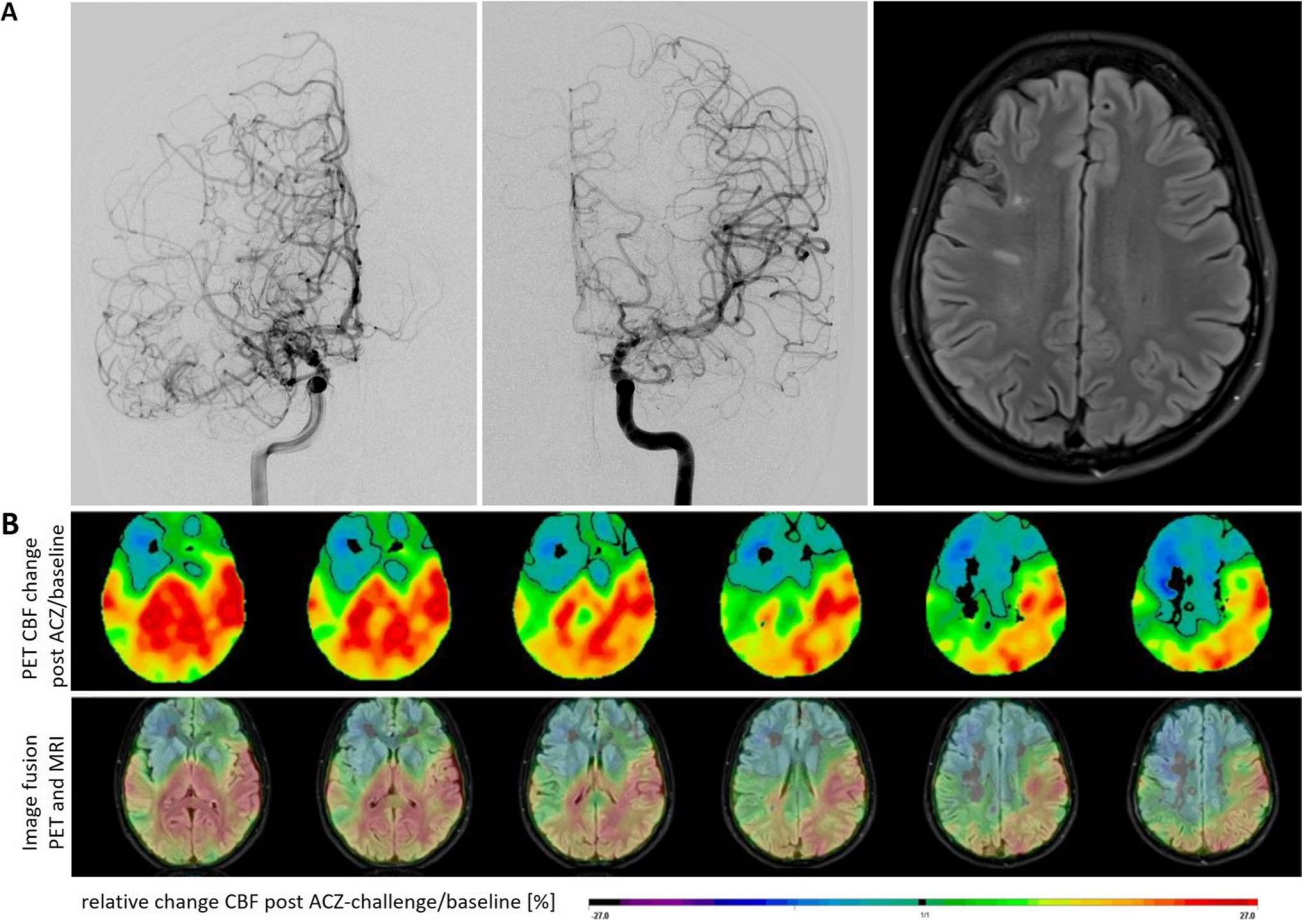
A upper row: Exemplary case of a 22-year-old female, right-handed, Caucasian patient with MMA at initial presentation after first manifestation with word-finding difficulties and short-term memory impairment. Neuropsychological testing revealed DCS with 3 of 4 abnormal test results. The BDI-II yielded 42 points, consistent with severe depression. The patient showed abnormal values in all subscales of the SCL-90-R and SF-36. Left: selective visualization of right ACI on angiography showing typical “puff of smoke” of as a correlate of a fine basal collateral network caused by occlusion of the MCA and stenosis of the A1 segment. Middle: Angiography of left ACI with hypoplastic A1 segment. Right: MRI T2 FLAIR sequence showing only small older infarctions in the right MCA territory. B lower row: Preoperative PET imaging with anatomical maps showing the perfusion reserve (in %) measured as difference between baseline measurement and after ACZ administration in H_2_^15^O PET
